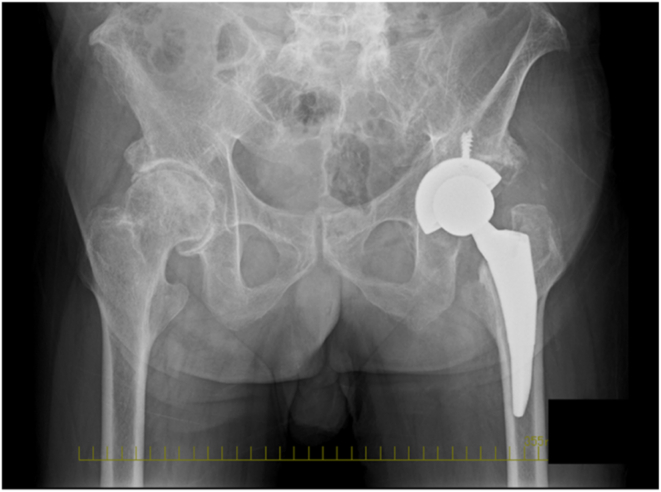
Postoperative pelvic radiograph.
